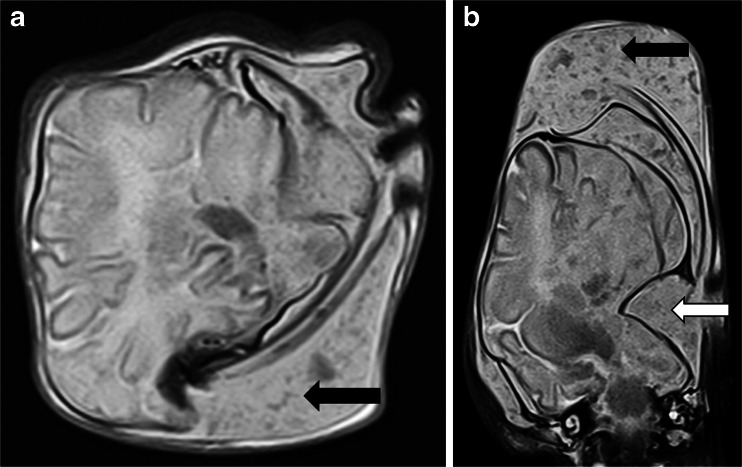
Severe head moulding. T2-weighted postmortem magnetic resonance imaging brain imaging of a 39-week gestation fetus with severe disruption of the skull following delivery, making both axial (a) and coronal (b) imaging difficult to interpret. There is soft tissue oedema (*black arrow*) as well as disruption of the skull, primarily along the sutures (*white arrow*). The intracranial appearances were normal at autopsy
